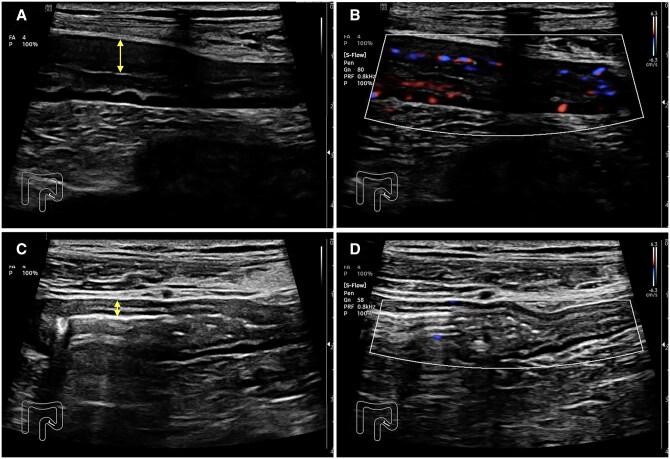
Intestinal ultrasound images demonstrating a longitudinal view of a sigmoid colon of a case who responded to intravenous (IV) cyclosporine. Before IV cyclosporine: B-mode image with increased BWT 6.2 mm (double-headed arrows) (A) and color Doppler signal with modified Limberg score 2 (B). Day 3 of IV cyclosporine: B-mode image with increased bowel wall thickness of 2.6 mm (double-headed arrows) (C) and color Doppler signal with modified Limberg score 0 (D). IV, intravenous; BWT, bowel wall thickness.
